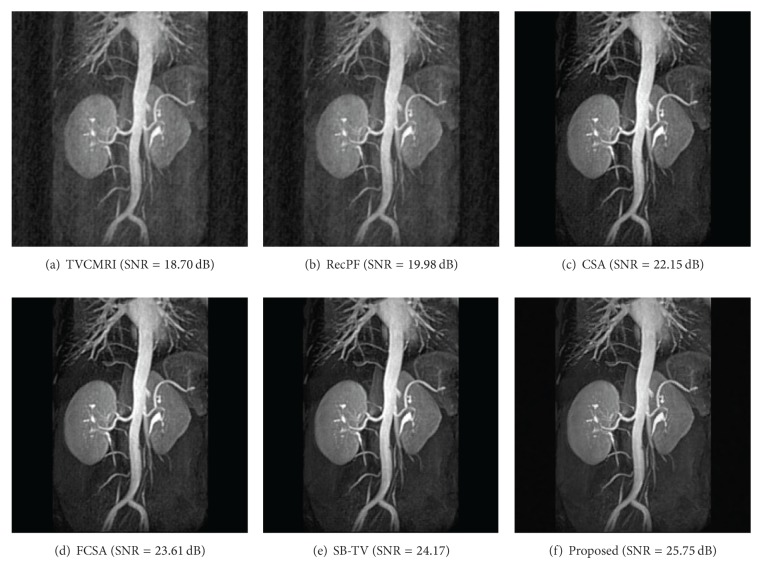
Artery MR image reconstruction from 20% samples (Gaussian noise case).
